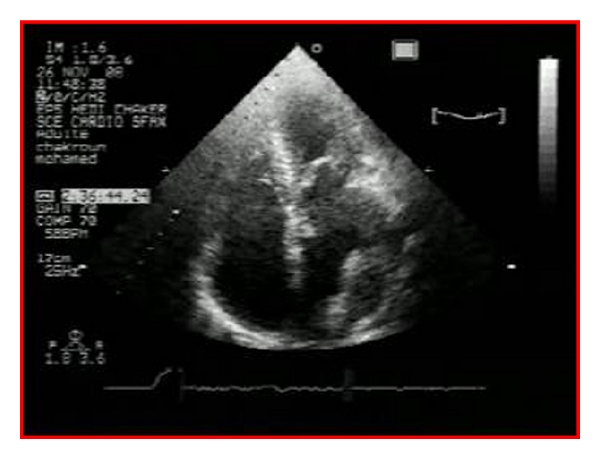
Transthoracic echocardiography: 4 chambers view showing a well-circumscribed mass in the let atrium.
